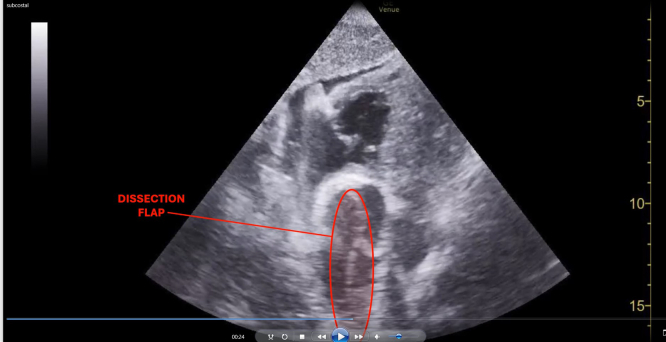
Subxiphoid point-of-care ultrasound showing ascending aorta with a dissection flap.
